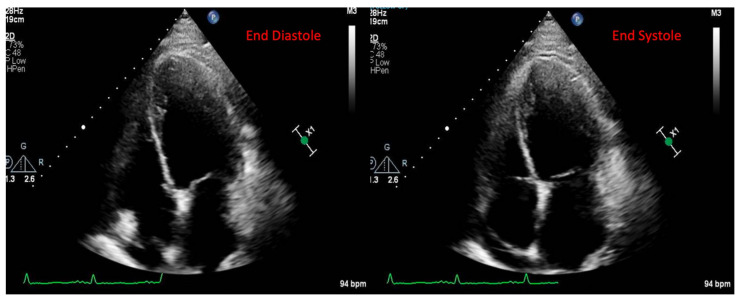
Echocardiogram images from the clinical patient. Transthoracic echocardiogram apical 4-chamber images (shown at end diastole and end systole). Severely decreased left ventricular ejection (30%). Mild right ventricular dysfunction. Small pericardial effusion.
